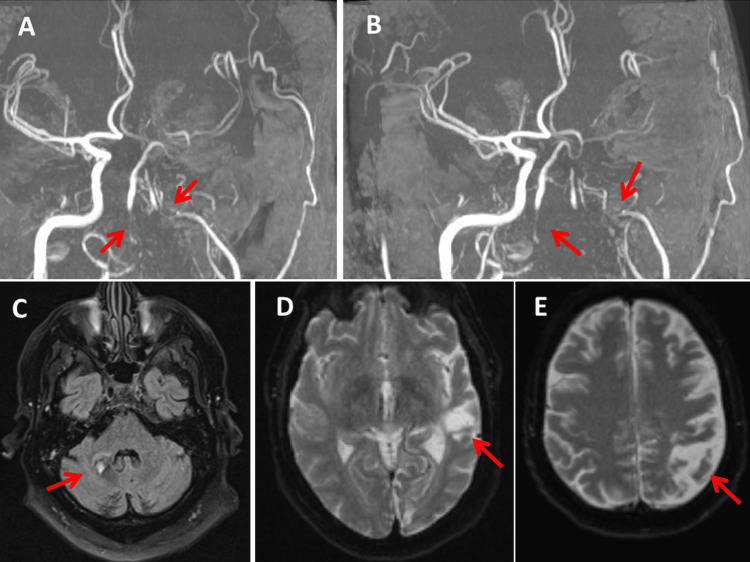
MRI of the index patient’s brain at the age of 54, with stenosis of the basilar artery and occlusion of the distal internal carotid artery (A and B) (red arrows) and old ischemic lesions in the left posterior cerebral artery and the territories of the left middle cerebral artery (D and E) (red arrows). MRI: magnetic resonance imaging
